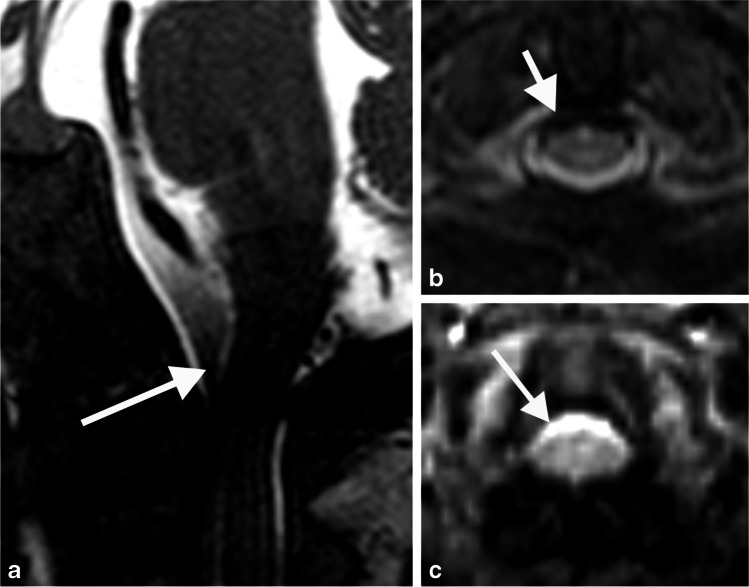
The effect of cerebrospinal fluid (CSF) flow void on achondroplasia foramen magnum score (AFMS): Sagittal (a) and axial (b, c) magnetic resonance images of the craniocervical junction in a 4-month-old girl with achondroplasia. Normal high signal CSF is seen above and below the foramen magnum. CSF flow void (*arrows*) anterior to the spinal cord is indistinguishable from the cortex of adjacent bone on T2 sequences (a, b). In contrast, CSF signal (*arrow*) is clearly present on the gradient echo sequence (c). CSF flow void (a, b) may lead to the assignment of a higher AFMS score than is warranted
